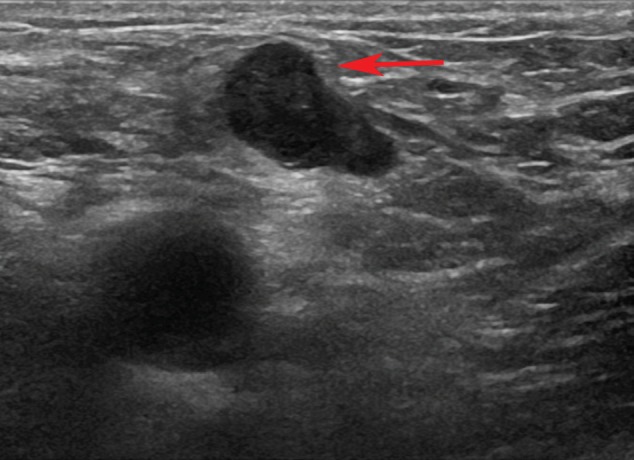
16-month follow-up US. The ultrasound exam shows a normal-sized lymph node in the right groin. It was considered suspicious because it has an asymmetric shape and cortical bulging (arrow).
